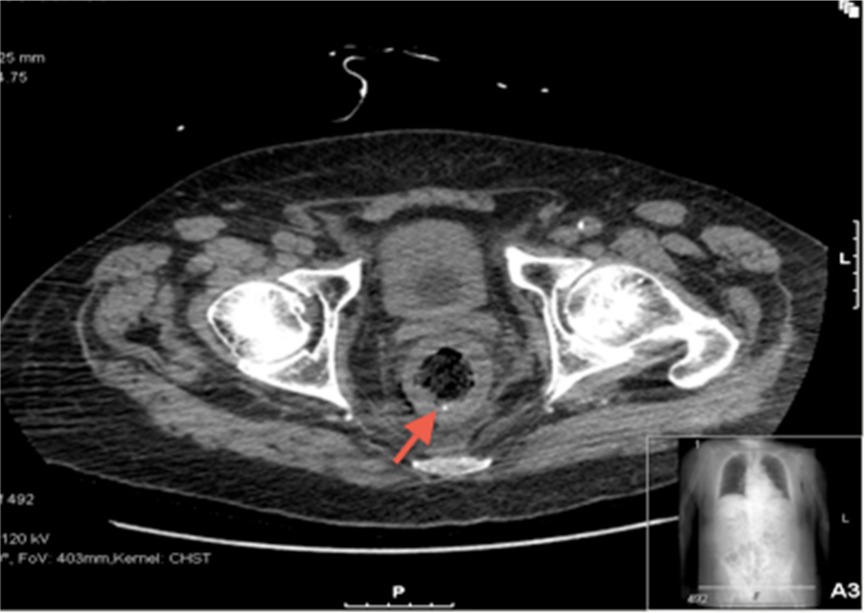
Computed tomography (CT) image of the lower abdomen. Red arrow shows concentric wall thickening of the rectum and fat stranding of the perirectal fascia.
